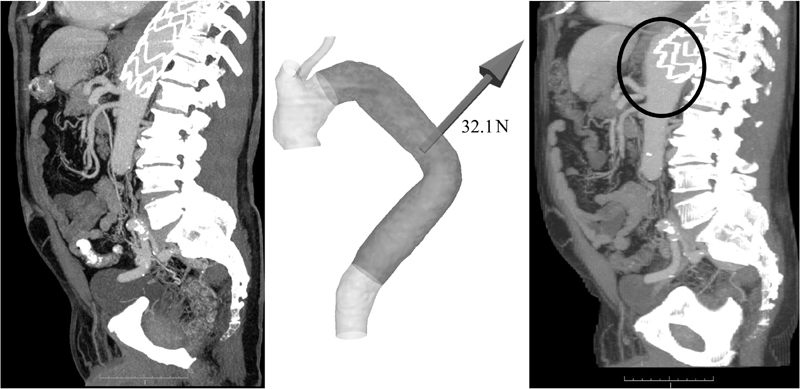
Displacement force and Type Ib endoleak in patient 4. On the left, a sagittal CT image of the postoperative situation is shown. In the middle, the postoperative model with the calculated displacement force vector is presented. On the right, a sagittal CT image at 1-year follow-up is shown, where proximal migration of the stent graft and resulting Type Ib endoleak was found (marked with circle).
